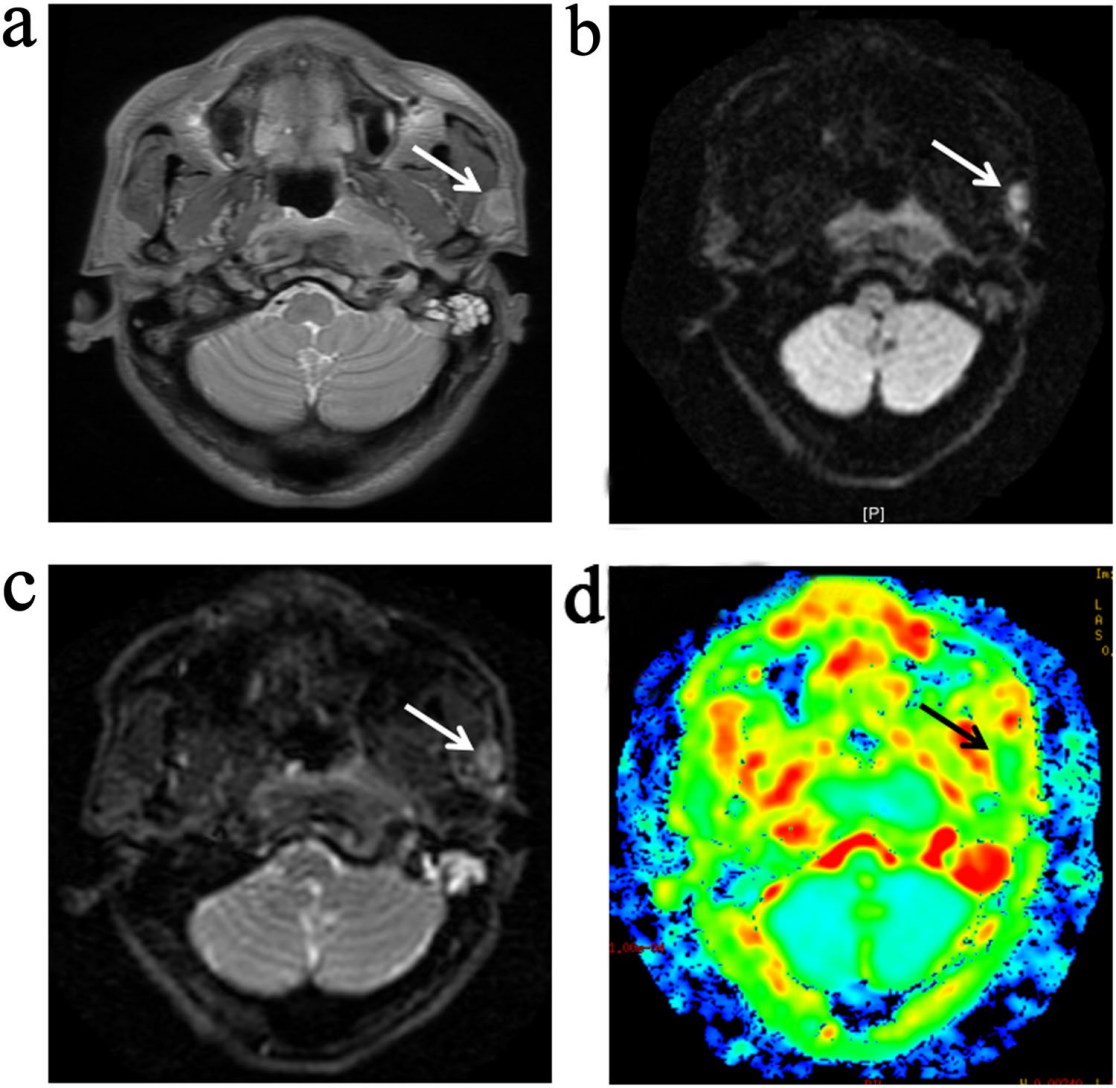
Transverse T2-weighted MR images of a benign PLN (a) (arrow). Axial diffusion-weighted MR images of PLN (arrows) show relatively reduced signal intensity achieved at *b* = 0 s/mm^2^ (b) and *b* *=* 800 s/mm^2^ (c). Consequently, the lesion shows hyperintensity on the ADC map (d).
